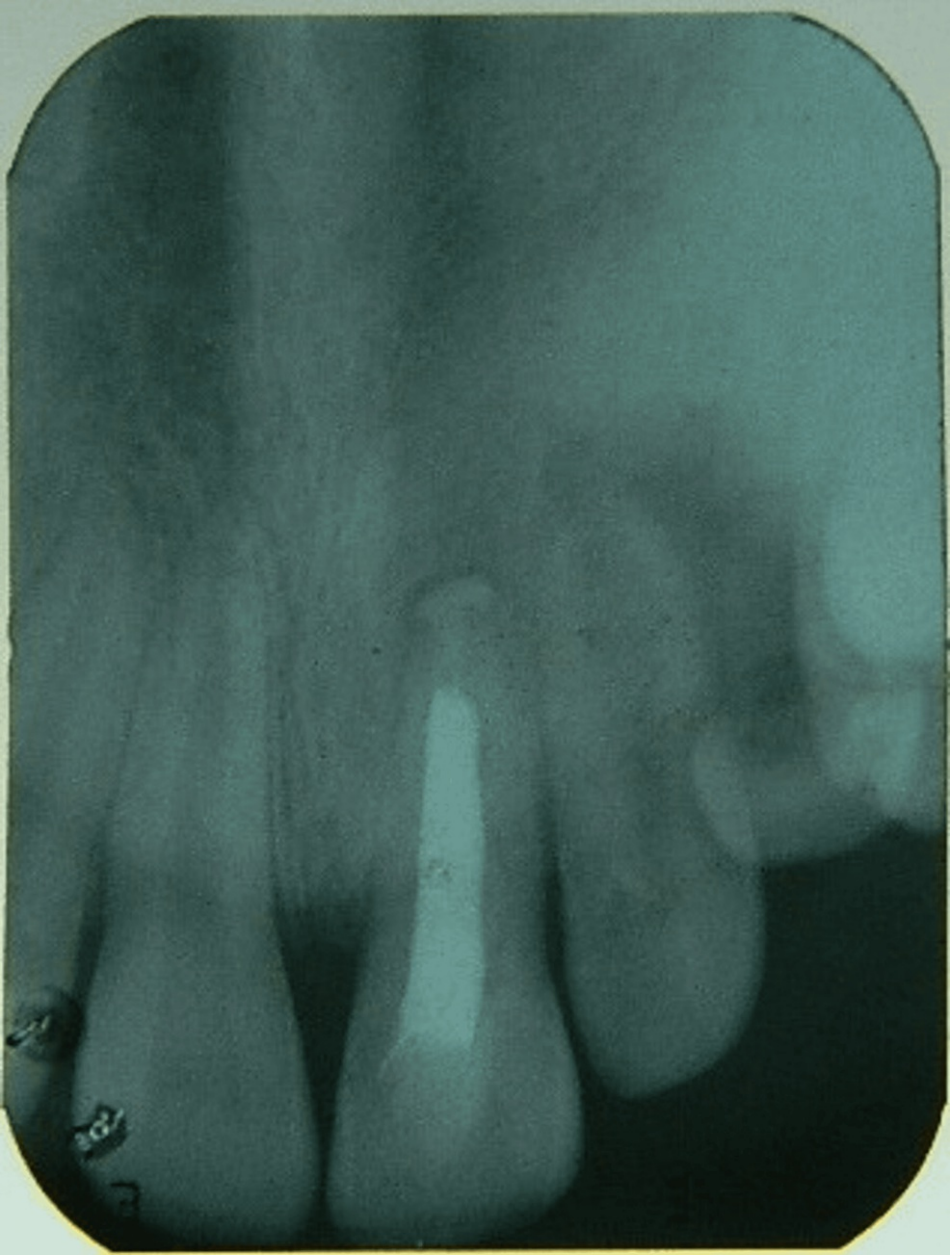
Post-operative IOPA (12 months follow-up) showing partial obliteration of the periodontal space IOPA: intraoral periapical
